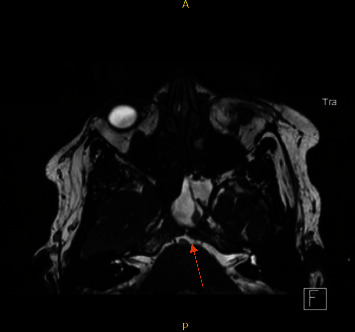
MRI skull base with IV contrast axial cut showing fluid accumulation in sphenoid communicating with clival defect (see arrow).
